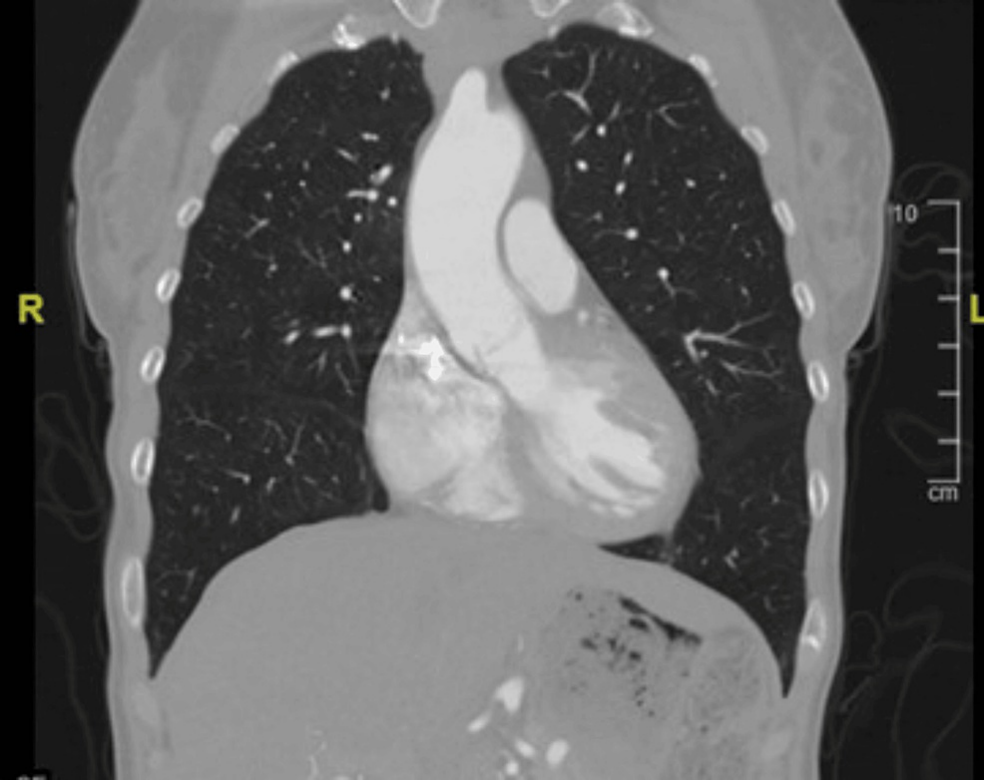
CT angiogram of the chest with contrast: No evidence of pulmonary emboli, aortic aneurysm, or dissection. Dense calcifications are present in the coronary arteries.
